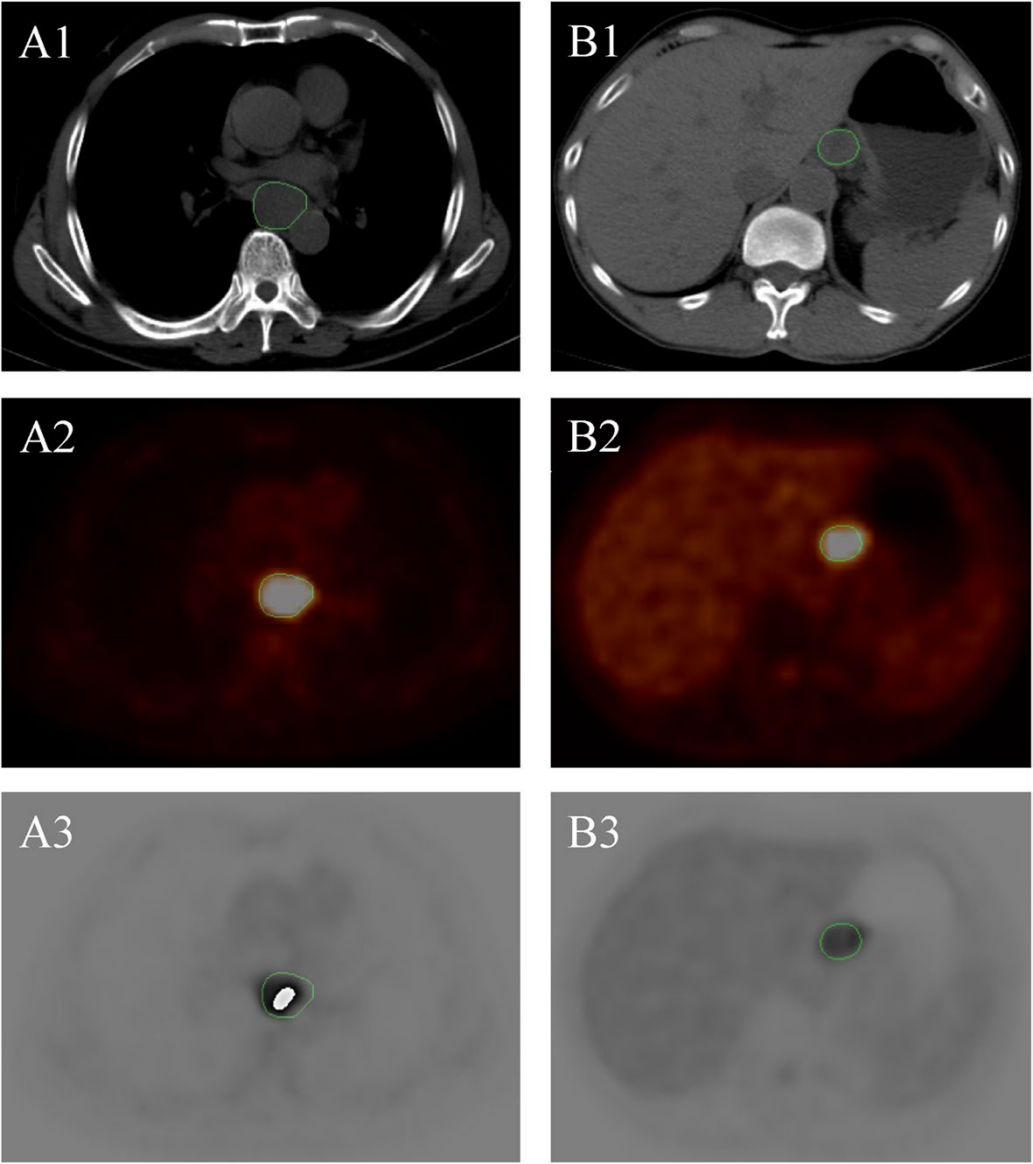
Demonstration of typical target segmentation of esophageal cancer. Forty percent threshold of 76-year-old man ESCC patient’s SUVmax: A1 CT image; A2 PET image; A3 fused CT and PET image. Threshold of 65-year-old man ESCC patient’s SUVmax: B1 CT image; B2 PET image; B3 fused CT and PET image
